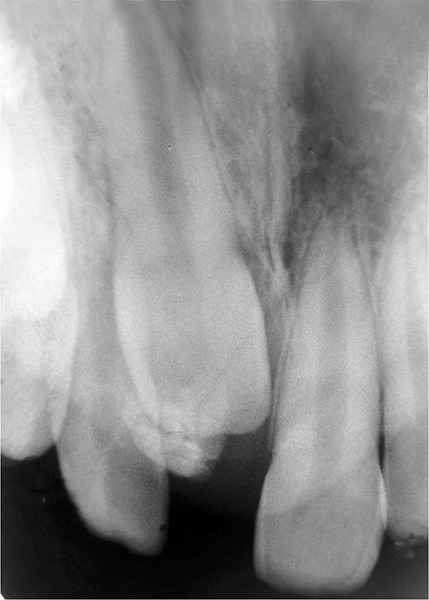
Periapical radiograph showing unerupted maxillary left central incisor with fully formed roots
